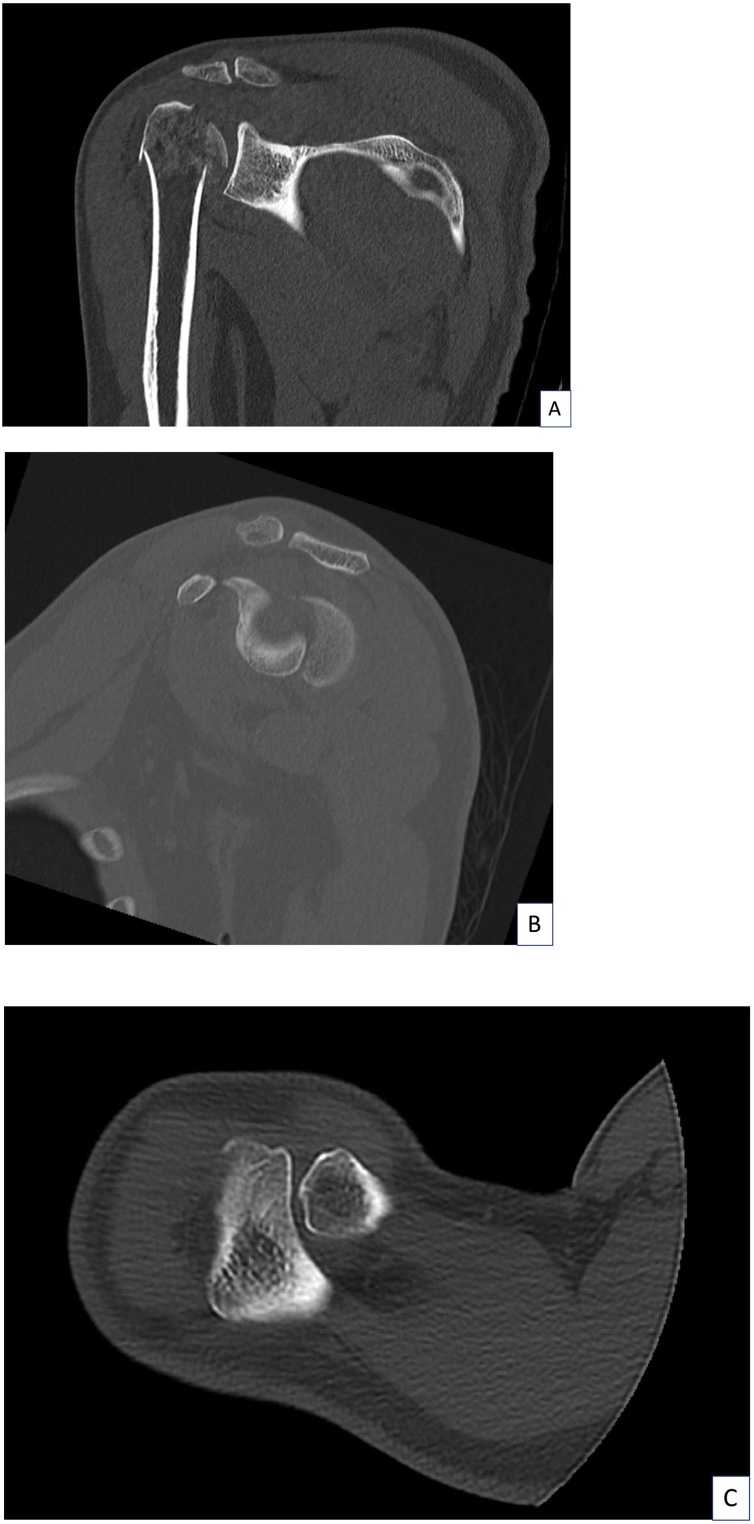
Initial computed tomography: (A) coronal oblique view, (B) sagittal view, and (C) axial view.
